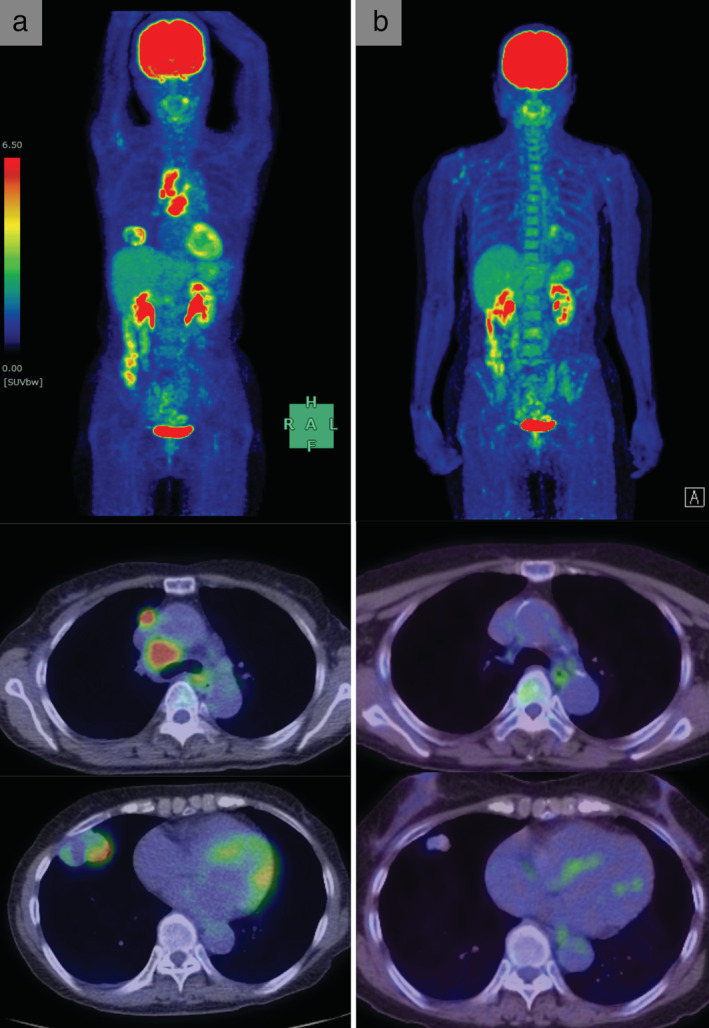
Fluorine‐18‐deoxyglucose (FDG)‐positron emission tomography imaging. (a) Before treatment. FDG accumulated in primary tumor and mediastinal lymph node metastasis. (b) After chemotherapy. FDG accumulation was only slightly observed at the primary tumor site.
